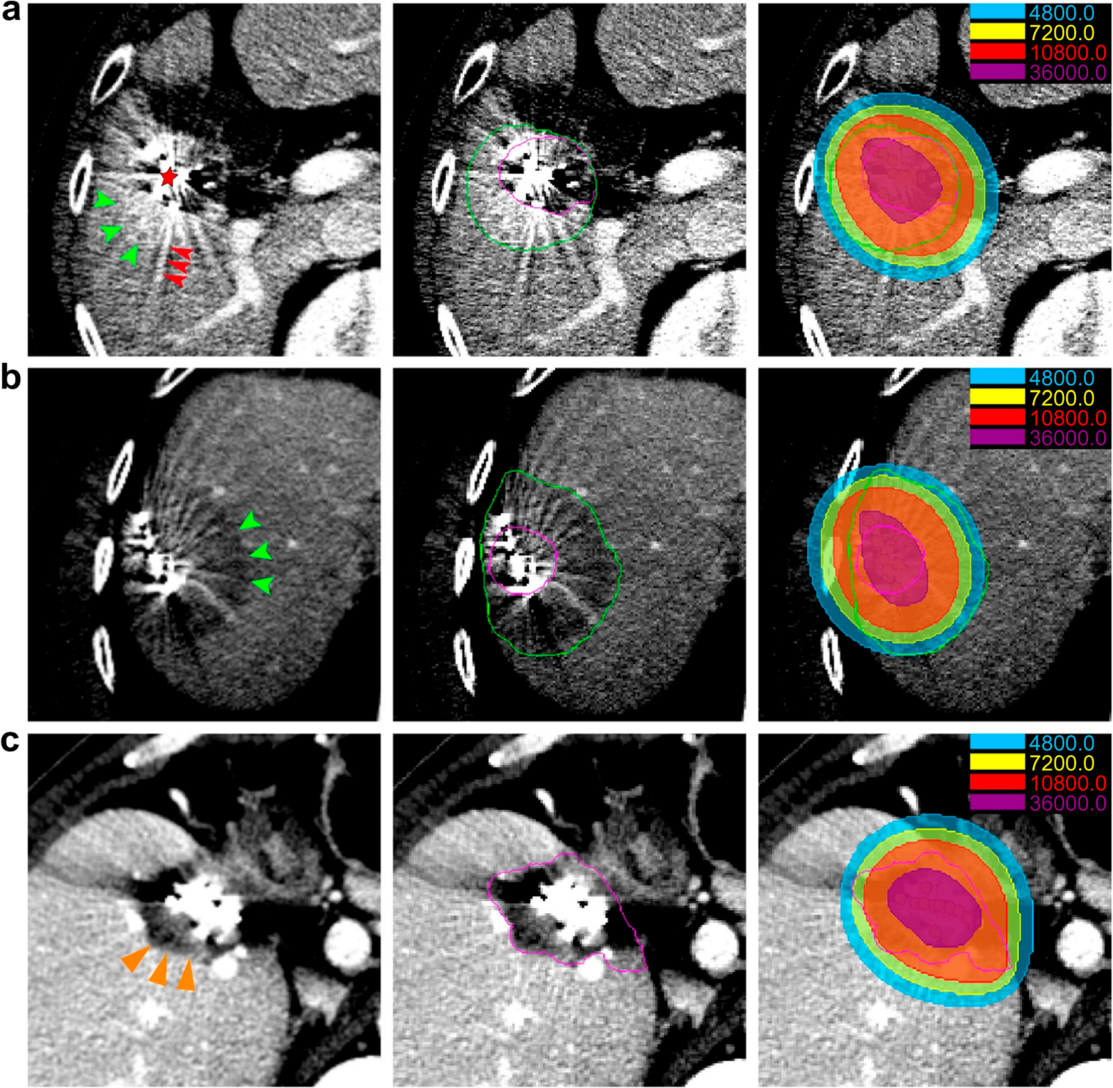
Peritumoral parenchymal abnormalities after brachytherapy on enhanced CT. A high- (a) and low-density (b) peritumoral reaction (green arrows) and normal peritumoral parenchyma (c) on enhanced CT are shown (left). The red star (a) marks the seeds, the red arrow refers to artifacts, and the orange arrow (c) refers to the progressive lesion. The lesion (purple) and reaction area (green) were delineated (middle). The isodose lines were calculated and drawn, which approximated the shape of the reaction (right). The doses represented by different colors are shown. Unit: cGy
